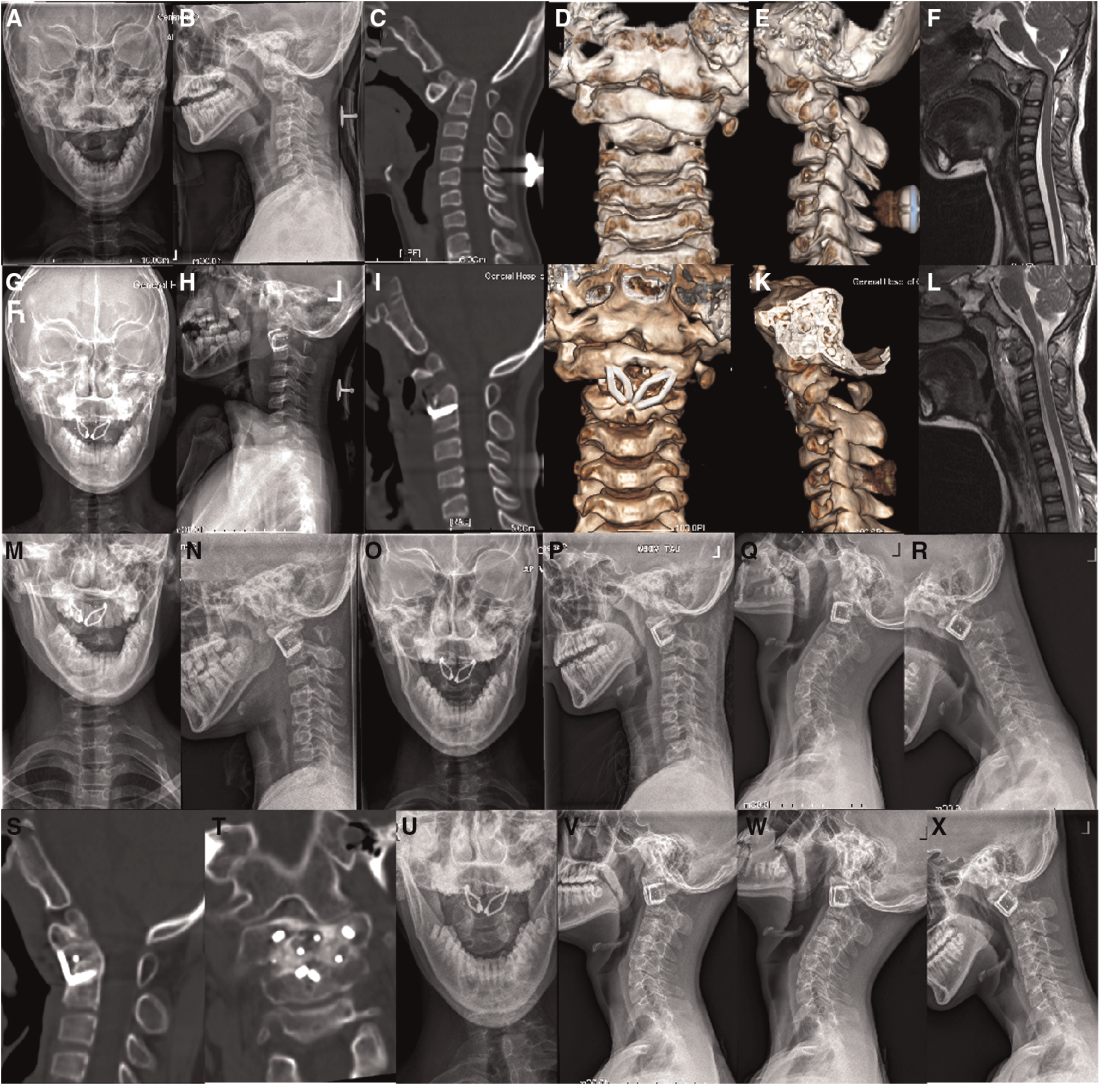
A 12-year-old boy, who was diagnosed IAAD with old odontoid fracture, underwent transoral intraarticular cage distraction and fusion with C-JAWS staple fixation. (A–E) Preoperative cervical x-rays and CT scans with three-dimensional reconstruction showed evidence of IAAD with old odontoid fracture. (F) Preoperative Sagittal MRI revealed compression of the spinal cord. (G–K) Cervical x-rays and CT scans with three-dimensional reconstruction performed at 1 week after revision surgery showed satisfactory reduction and good placement of fixation and cages. (L) Postoperative sagittal MRI showed a desirable decompression of the spinal cord. (M,N) Cervical x-rays at 3-month follow-up showed stable fixation. (O–R) Cervical x-rays at 6-month follow-up showed stable fixation without loss of reduction. (S,T) CT scans at 6-month follow-up revealed a solid bone fusion. (U–X) Cervical x-rays at last follow-up showed good C1–C2 sequence.
